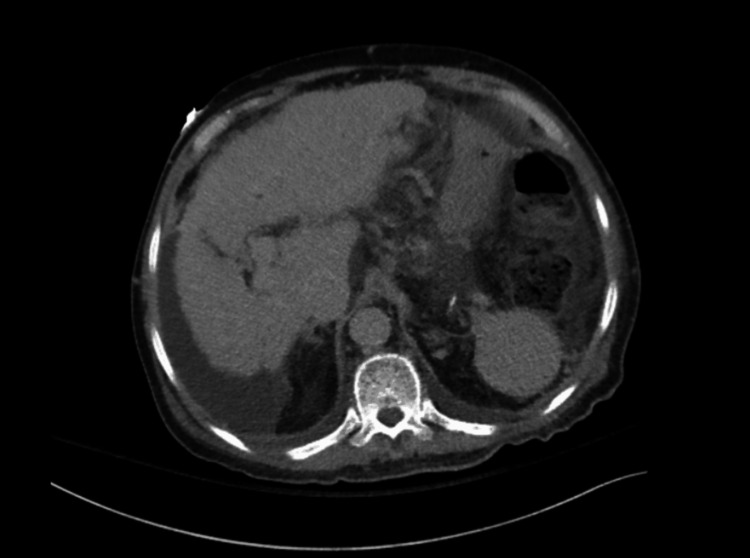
Contrast-enhanced CT of the abdomen and pelvis demonstrating an atrophic liver with surface nodularity consistent with cirrhosis, without intrahepatic bile duct dilation or arterial enhancing hepatic lesions.
